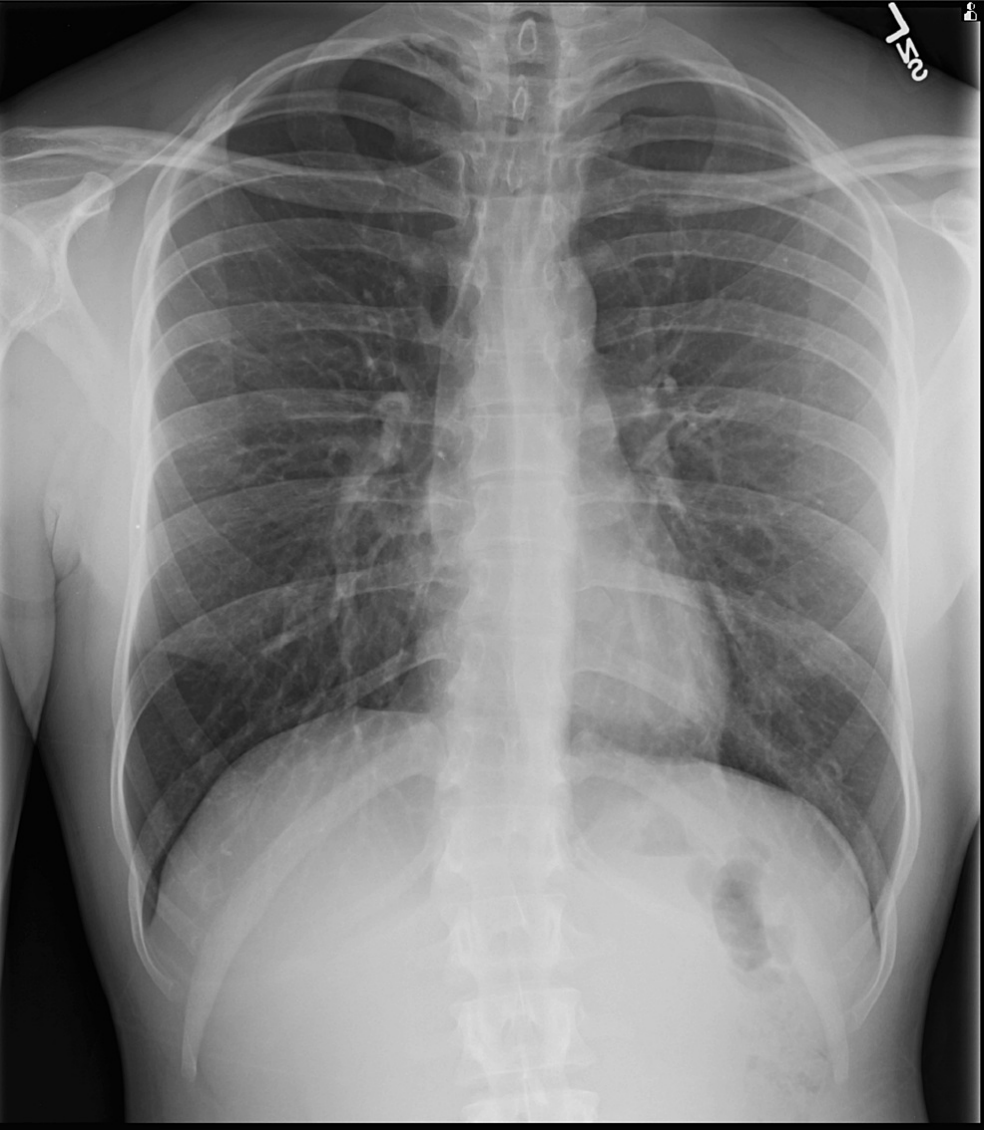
Chest X-ray showing resolution of pneumothorax.
